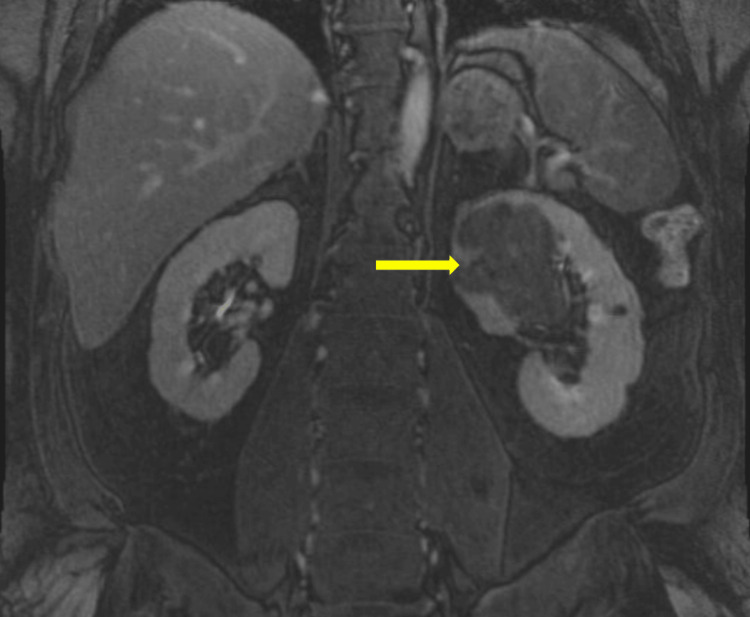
MRI T1 of the left renal mass (yellow arrow) invading the collecting system
